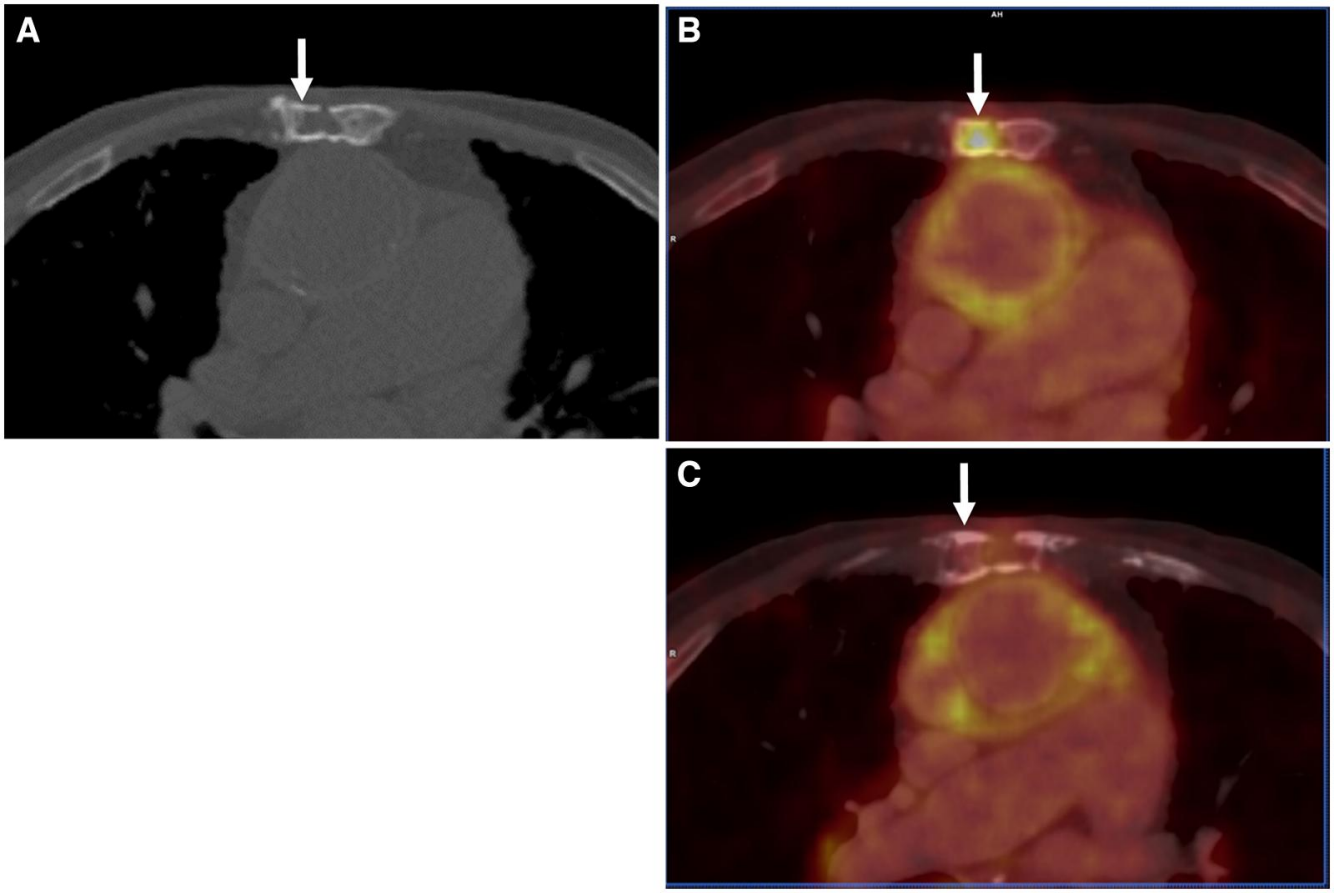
Tuberculous osteomyelitis of the chest wall in an 83-year-old man who had prolonged fever. Axial (A) CT and (B) ^18^F-FDG PET/CT fusion images show a destructive osteolytic lesion in the sternum that has intense metabolic activity (white arrows). (C) Axial PET/CT fusion image taken 8 months later following medical treatment shows regression of uptake in the lesion (white arrow). (Courtesy of Dr Tawika Kaewchur, Chiang Mai University, Thailand.)
